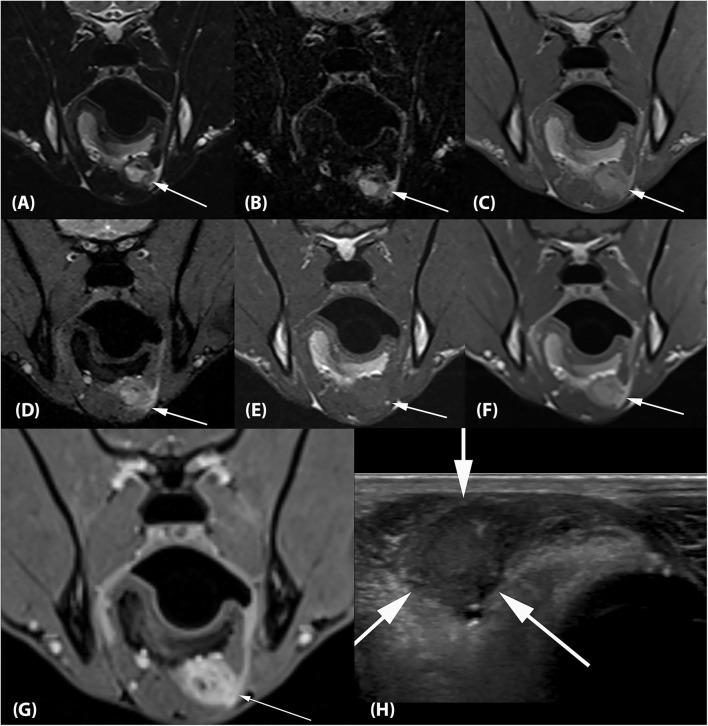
(A) Transverse T2-weighted, (B) T2-FLAIR, (C) PD-weighted, (D) T2*-weighted GRE, (E) T1-weighted pre contrast, (F) T1-weighted post contrast and (G) T1-weighted Volume Interpolated GRE images with fat saturation (“VIBE”) at the level of the mandibular angle. There is an ~2.0 × 1.3 × 1.8 cm (L × H × W) ovoid lesion associated with the left side of the tongue base with imaging characteristics identical to the lesion associated with the left medial pterygoid muscle (arrows). (H) Transverse ultrasonographic image of the lesion at the tongue base which is hypointense, fairly homogeneous, and well-circumscribed (arrows). The impression of the lesion being right- rather than left-sided is artifactual and related to a change in tongue position between the MRI and ultrasound examination.
